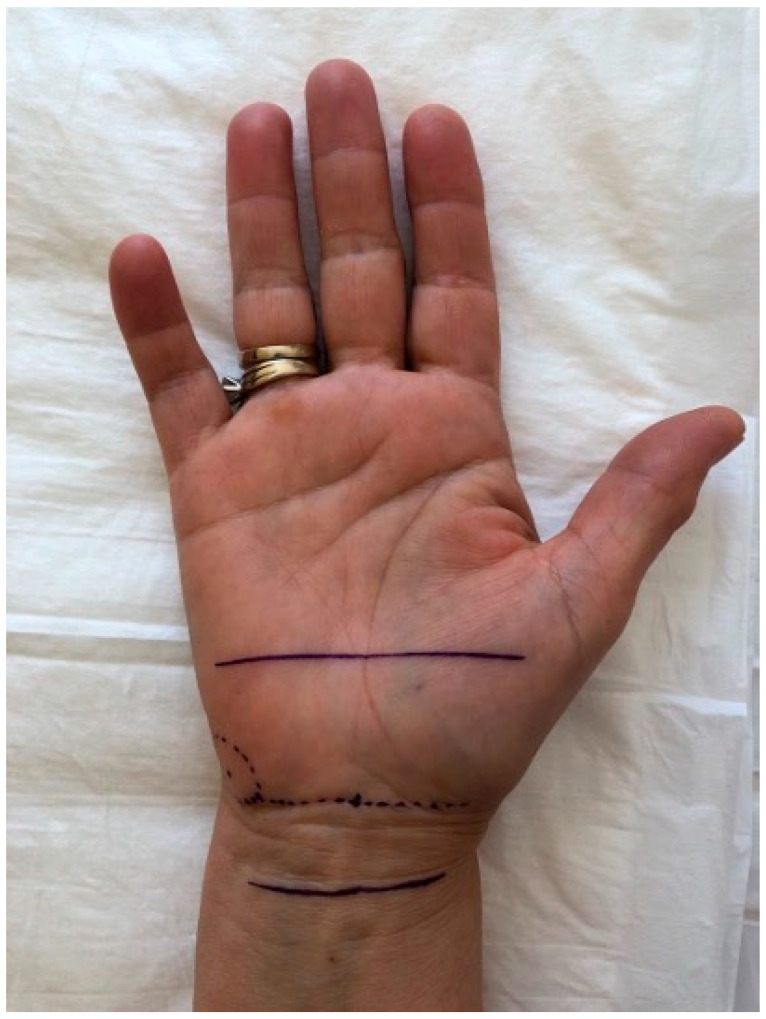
Surface anatomical landmarks for standardized sonographic evaluation of the median nerve in carpal tunnel syndrome. The central line (P) indicates the level of the pisiform bone, which corresponds to the carpal tunnel inlet. The proximal line (P−2 cm) is located 2 cm above the pisiform and represents the forearm level. The distal line (P+3 cm) is located 3 cm below the pisiform and corresponds to the mid-carpal tunnel level. These landmarks assist in consistent probe placement and allow reproducible cross-sectional area measurements of the median nerve along its course.
